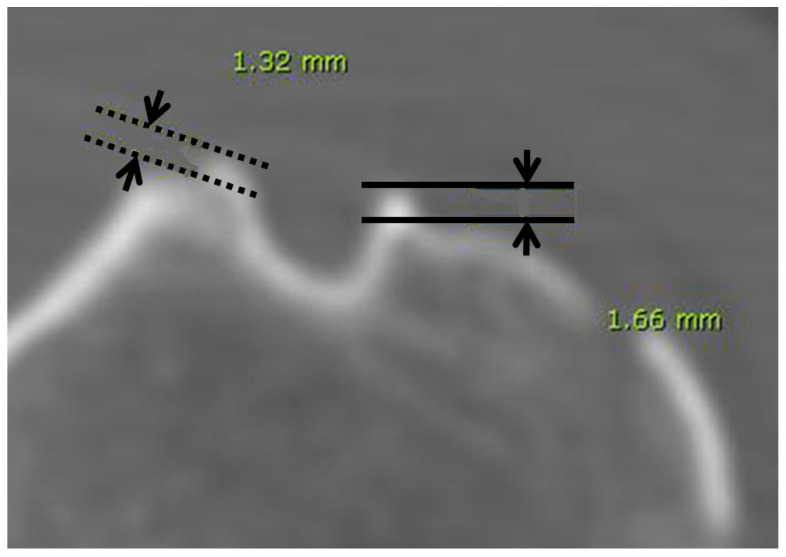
The axial CT image shows the bicipital spurs. The solid lines indicate the height of the lateral spur, while the dashed lines indicate the height of the medial spur.
